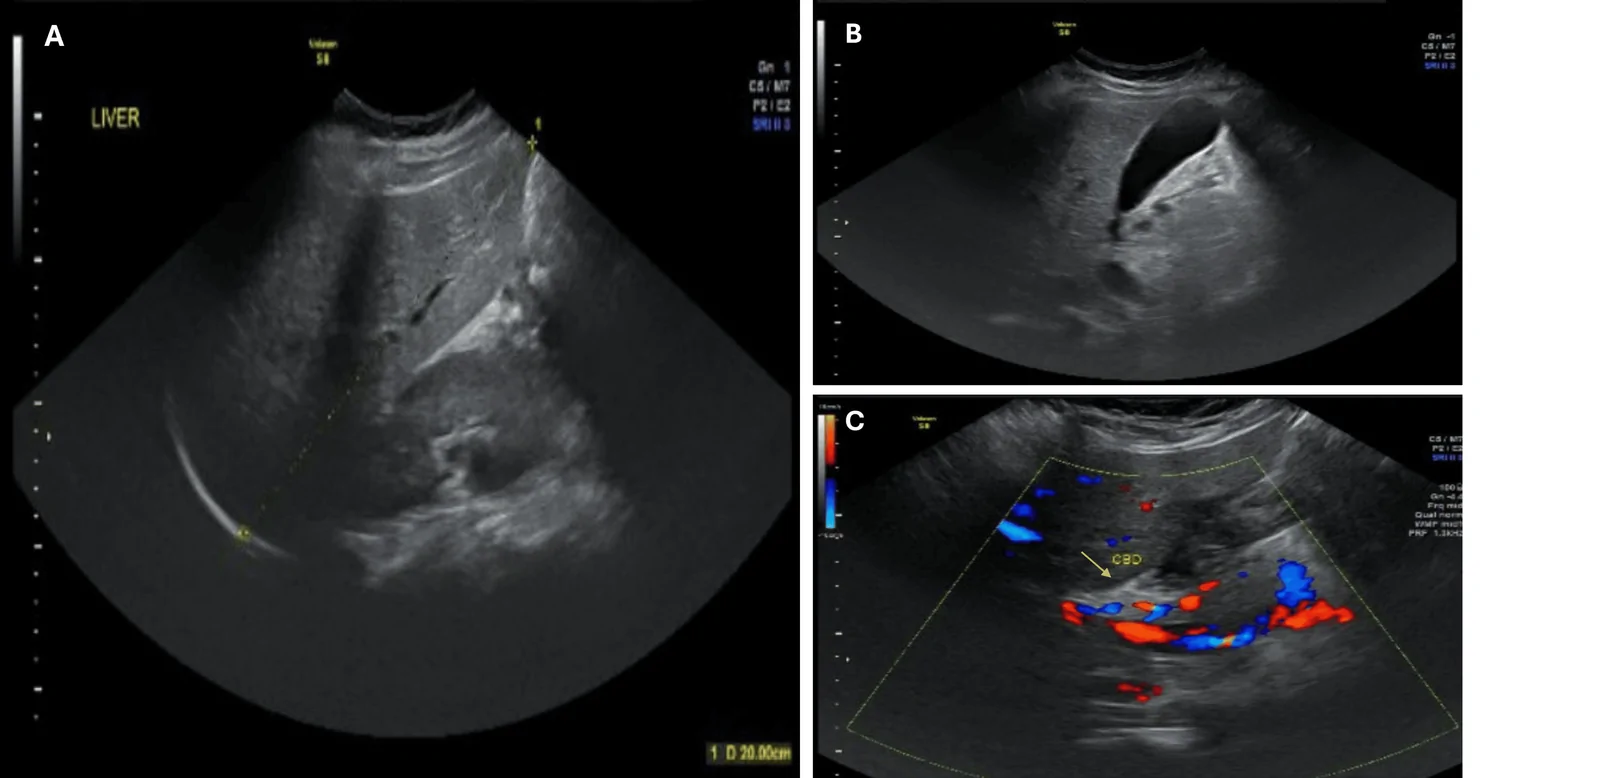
Abdominal ultrasonography demonstrating mild hepatomegaly without biliary dilatation or gallstones (A) Mild hepatomegaly (liver span approximately 20 cm) with preserved hepatic echotexture. (B) Normal gallbladder without gallstones or features of acute cholecystitis. (C) Color Doppler image demonstrating a nondilated common bile duct (arrow) without biliary obstruction.
